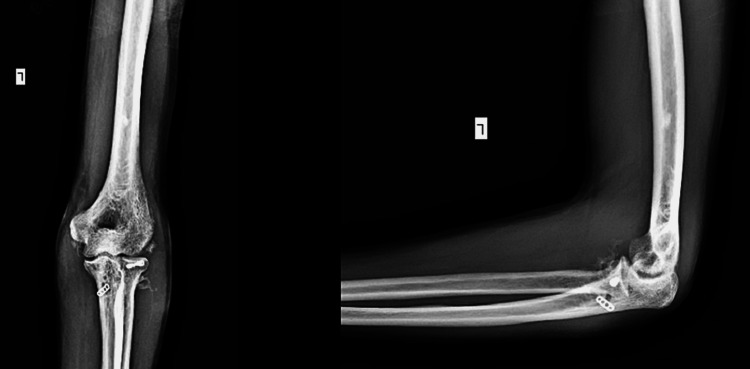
Eighteen months postoperative X-ray showing healed fracture of radial head and coronoid with no myositis
